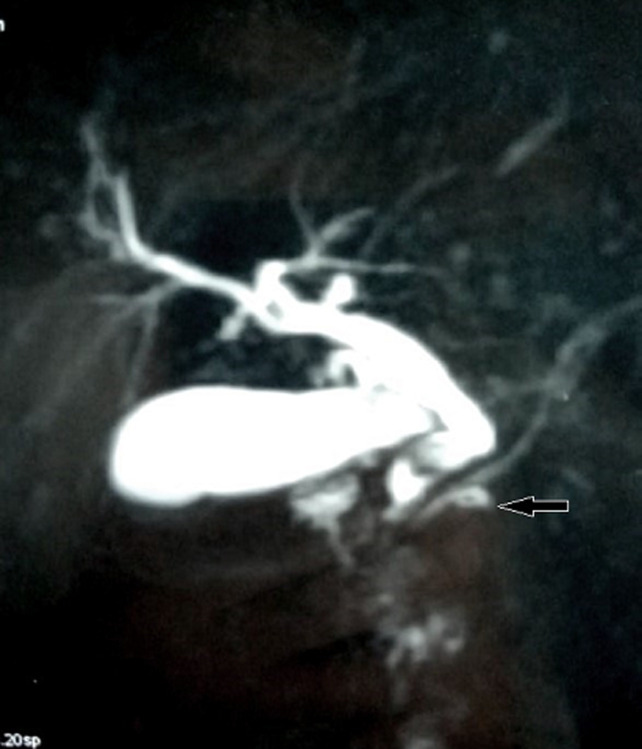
MRI showing a dilatation of the princpale biliary tract upstream of a lithiasis with the appearance of a loop at the level of the wirsung canal evoking an ansa pancreatica
